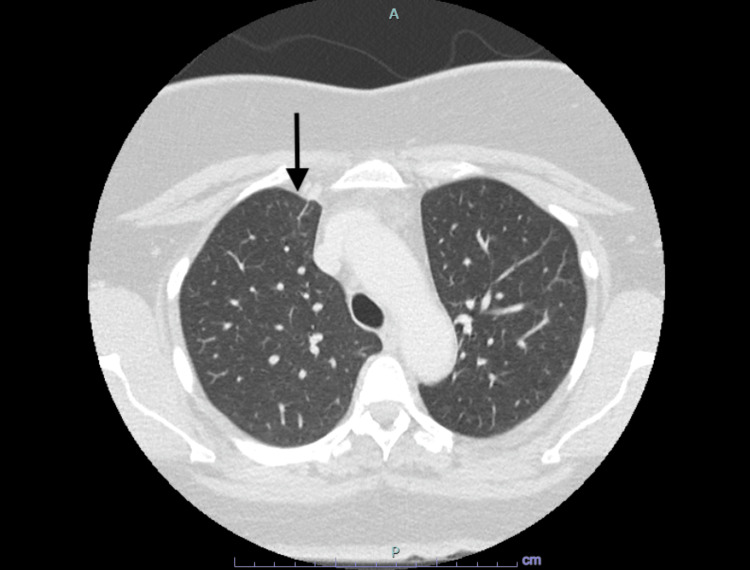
CT scan of thorax after 12 weeks of effective antibiotics showing resolving lung infection with resolved right upper lobe lesion. A: anterior, P: posterior.
